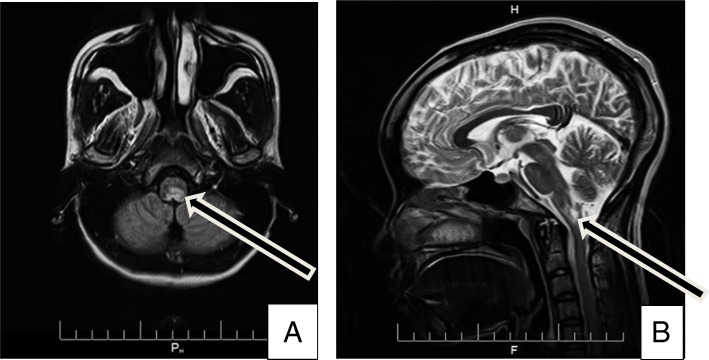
Axial Fluid Attenuated Inversion Recovery (a) and sagittal T2-weighted magnetic resonance imaging sequences demonstrate a bulbar left more lateralized lesion (arrows)
